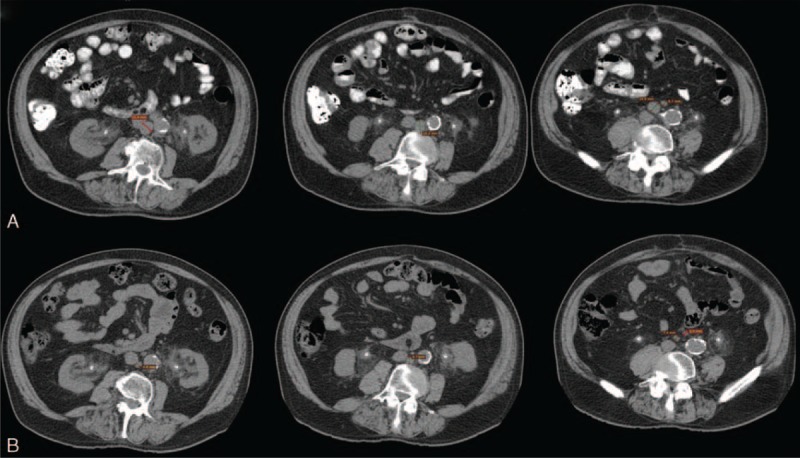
CT scan showing target lymphnodes at baseline (A) and after 6 months of treatment (B). CT = computed tomography.
